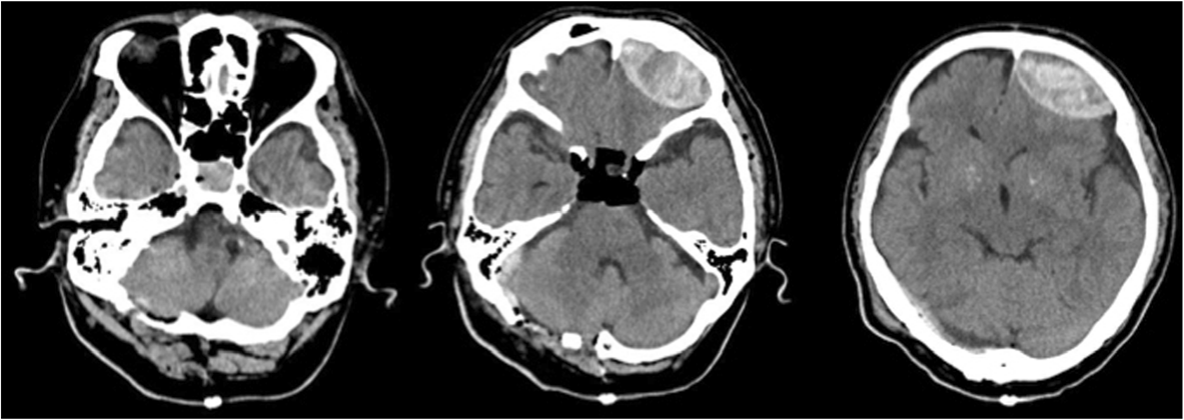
Head computed tomography after surgery. Computed tomography showed enlargement of the left frontal acute epidural hematoma
